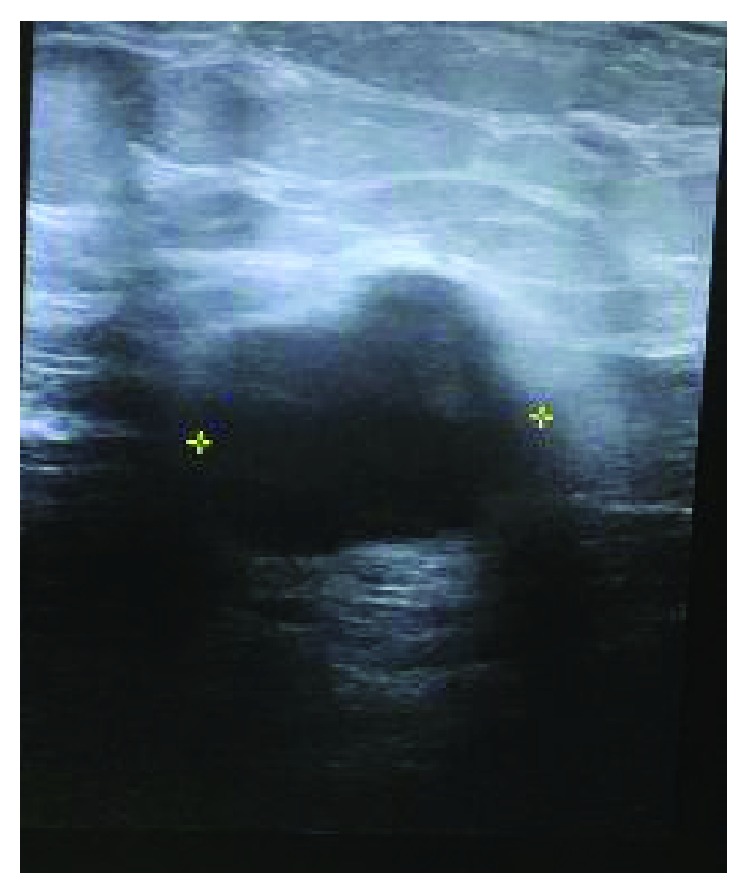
Sonogram of the left breast showing hypoechoic lesion.
